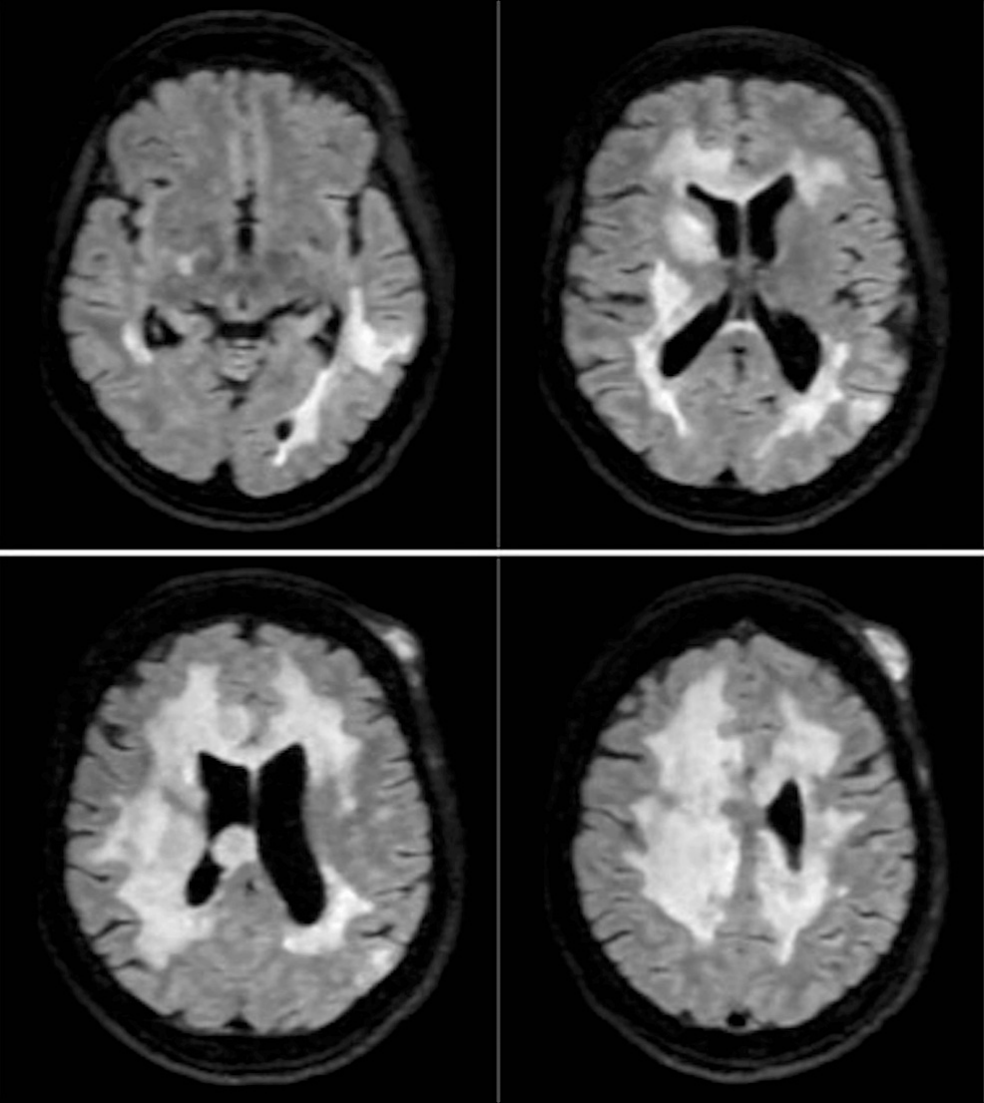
Axial view of MRI T2 FLAIR sequencing showing mass lesions within the supratentorial region bilaterally FLAIR: fluid-attenuated inversion recovery
